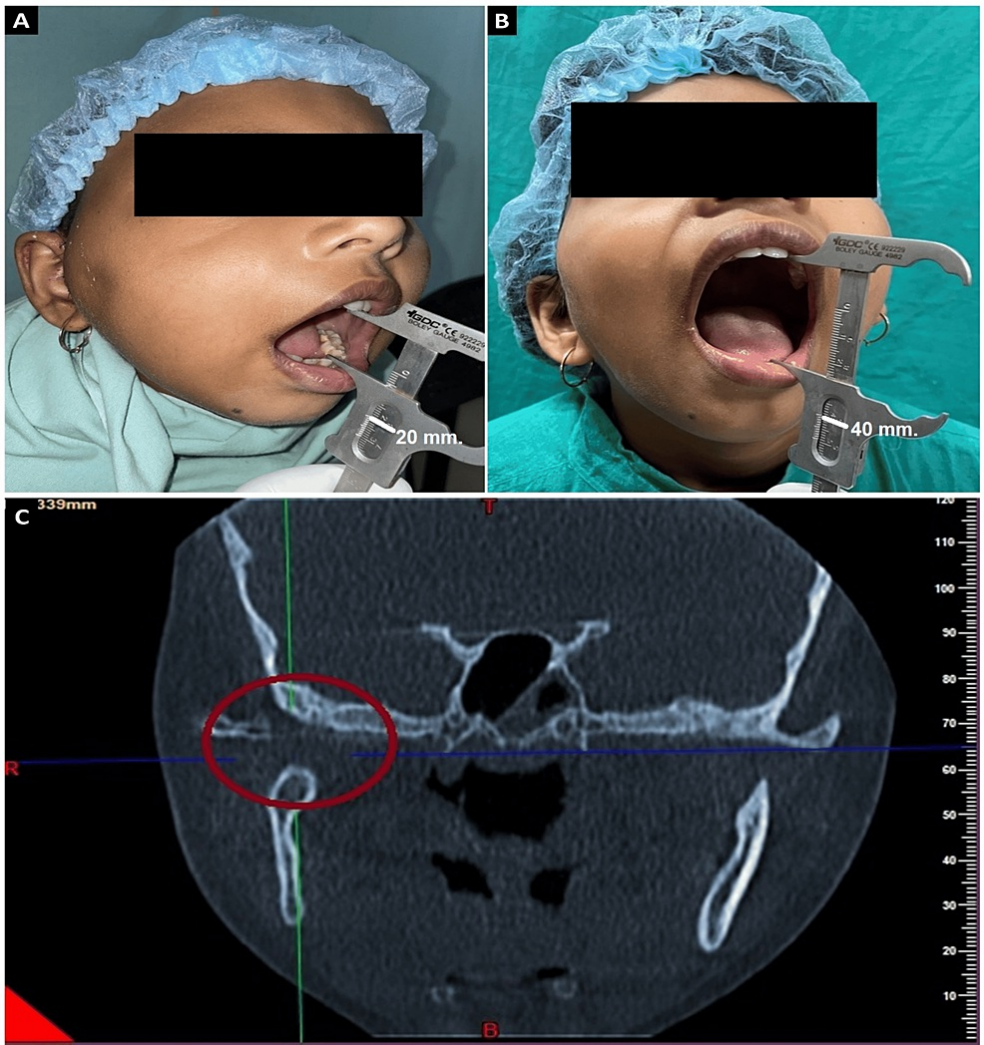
Post-operative evaluation of the patient at different follow-up periods (A) Observation of a 20 mm mouth opening at the one-week follow-up (vernier caliper reading marked with white marker) (B) Observation of a 40 mm mouth opening at the one-year follow-up (C) Coronal section obtained from cone beam computed tomography (CBCT), highlighting no heterotopic bone formation or re-ankylosis
